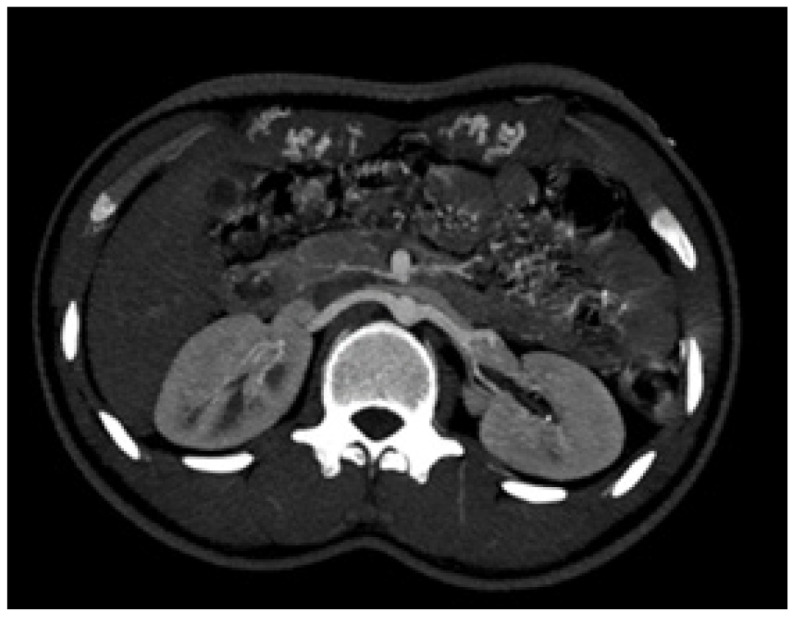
Computed tomography renal axial section showing normal renal arteries.
